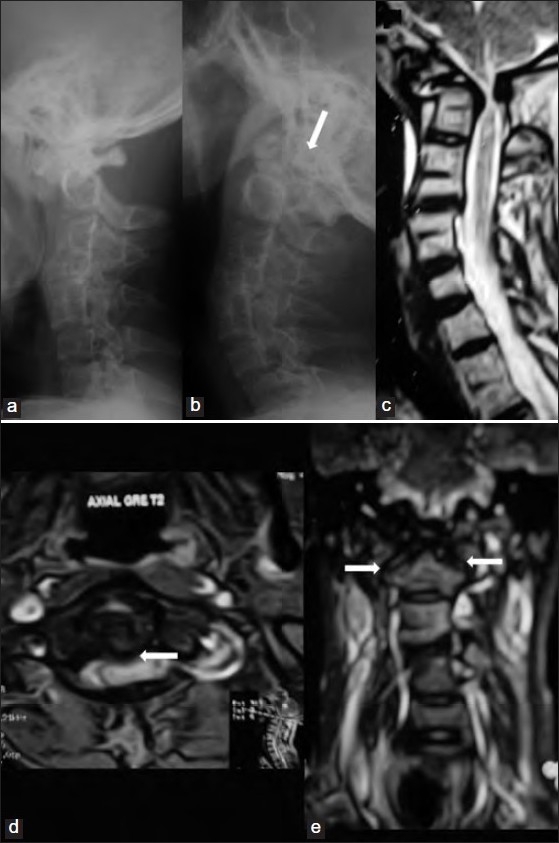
Lateral radiograph of craniovertebral junction in flexion (a) extension (b) and sagittal T2-weighted MR image (c) showing OO with Klippel-Feil anomaly (C3-4, C5-6 and thoracic 2-3 vertebral fusions) with retrolisthesis of C1 arch-os odontoideum complex (arrow) during neck extension (b). T2-weighted axial MR image (d) showing significant cord compression (arrow) with T2 hyperintense changes; and coronal image (e) showing the nearly symmetrical facet joint surfaces (arrows)
